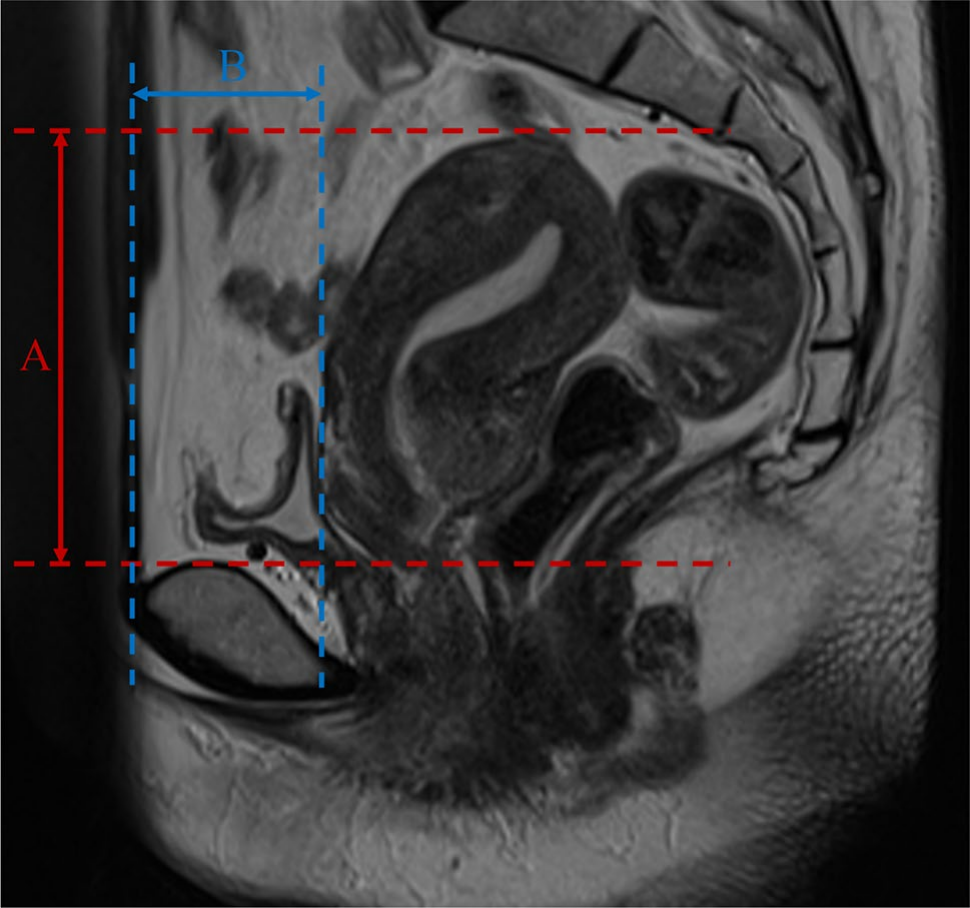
The definition of the distance to the boundary of the uterus in the median plane (sagittal plane through the midline of the body) on magnetic resonance images (an example of 1 patient, on a T2-weighted magnetic resonance image). A means the distance from the superior border of the fundus uteri to the superior border of the pubic symphysis. B means the distance from the anterior border of the uterus to the anterior border of the pubic symphysis
